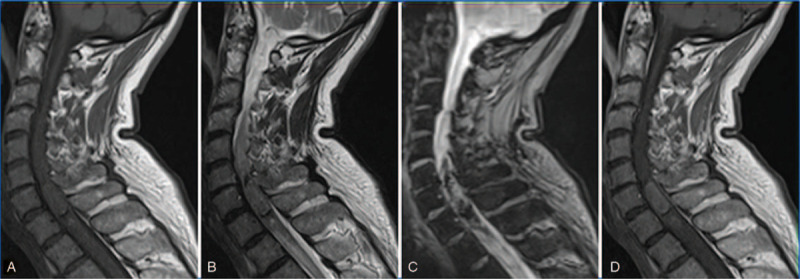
Cervical medullar magnetic resonance imaging, sagittal plane. The intra medullar lesion is hyperintense on T1-weighted sequence (A), isointense on T2-weighted sequence (B), presents signs of bleeding on T2^∗^-weighted sequence (hypointensity) (C) and homogeneous enhanced with gadolinium injection (D).
